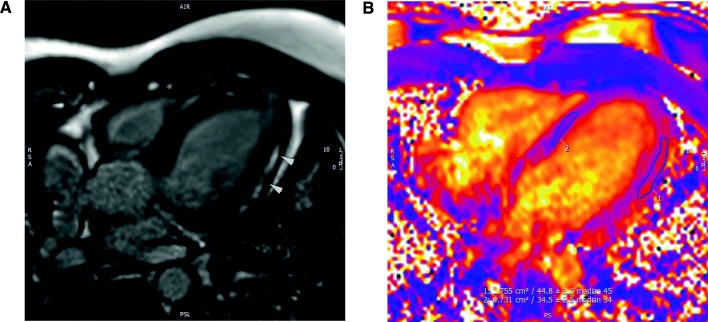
a LGE 4-chamber images showing the presence of an area of enhancement in the mid-wall portion of the lateral wall of the left ventricle (arrowheads). Heterogeneous, focal areas of LGE can also be identified in the septal wall. The localization of these areas of LGE are typical for patients with myocarditis and represent one of the main criteria for its diagnostic by MRI. b T2 parametric maps of the same patient in A, at the same 4-chamber slice position. The region-of-interest 1 in the lateral wall shows a T2 value of 44.8ms versus a T2 in the septum (ROI 2) of 34.5ms. The increased T2 identified in the lateral wall colocalizes with the LGE area seen in A and suggests the presence of edema/inflammation in comparison to an apparently normal area in the septum
